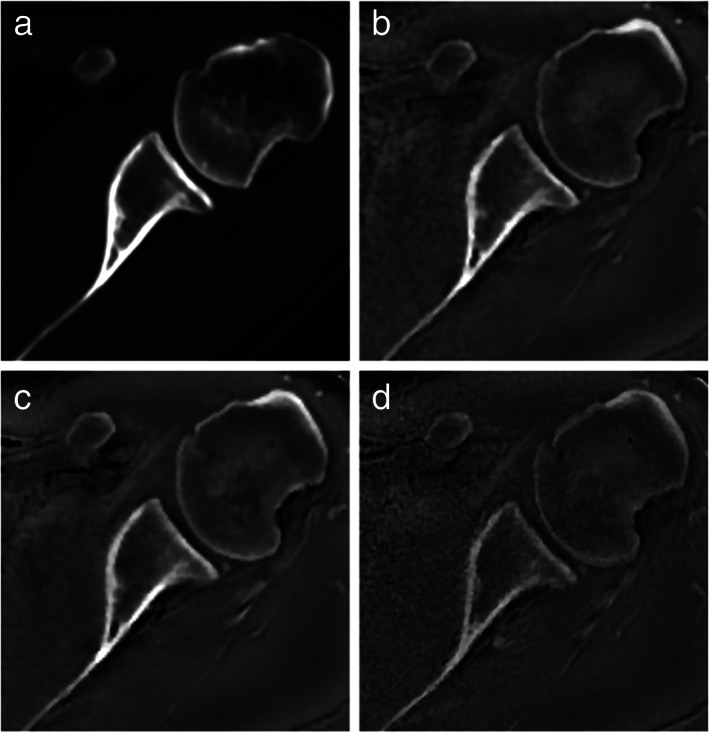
Axial computed tomography and zero echo time magnetic resonance imaging of left shoulder in a 38‐year‐old man. Axial images obtained by computed tomography (a) and zero echo time magnetic resonance imaging at 1.0 mm^3^ (b), 0.8 mm^3^ (c), and 0.7 mm^3^ (d) all show high‐contrast imaging of the osseous structures, including the glenoid and glenohumeral joint. Reprinted with permission from reference 19.
